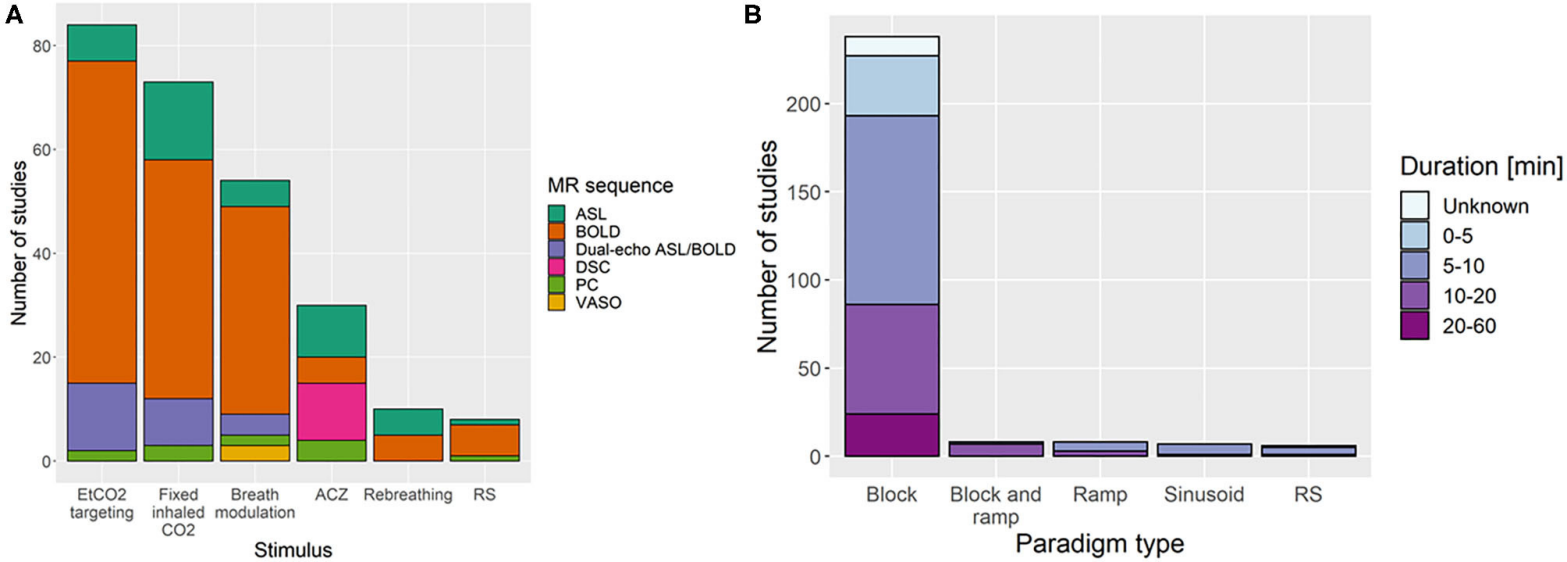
Distribution of the (A) stimuli with the associated MRI sequence and (B) paradigm types with associated total duration of the CVR experiment. In (A), the “breath modulation” stimulus includes breath-holding, paced breathing, and hyperventilation stimuli. ACZ, acetazolamide injection; RS, resting-state; BOLD, blood oxygen-level dependent; ASL, arterial spin-labelling; PC, phase contrast; DSC, dynamic susceptibility contrast; VASO, vascular space occupancy.
